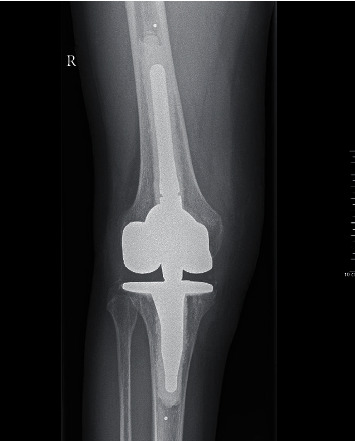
Postoperative radiographs showing the anteroposterior view of the right knee.
